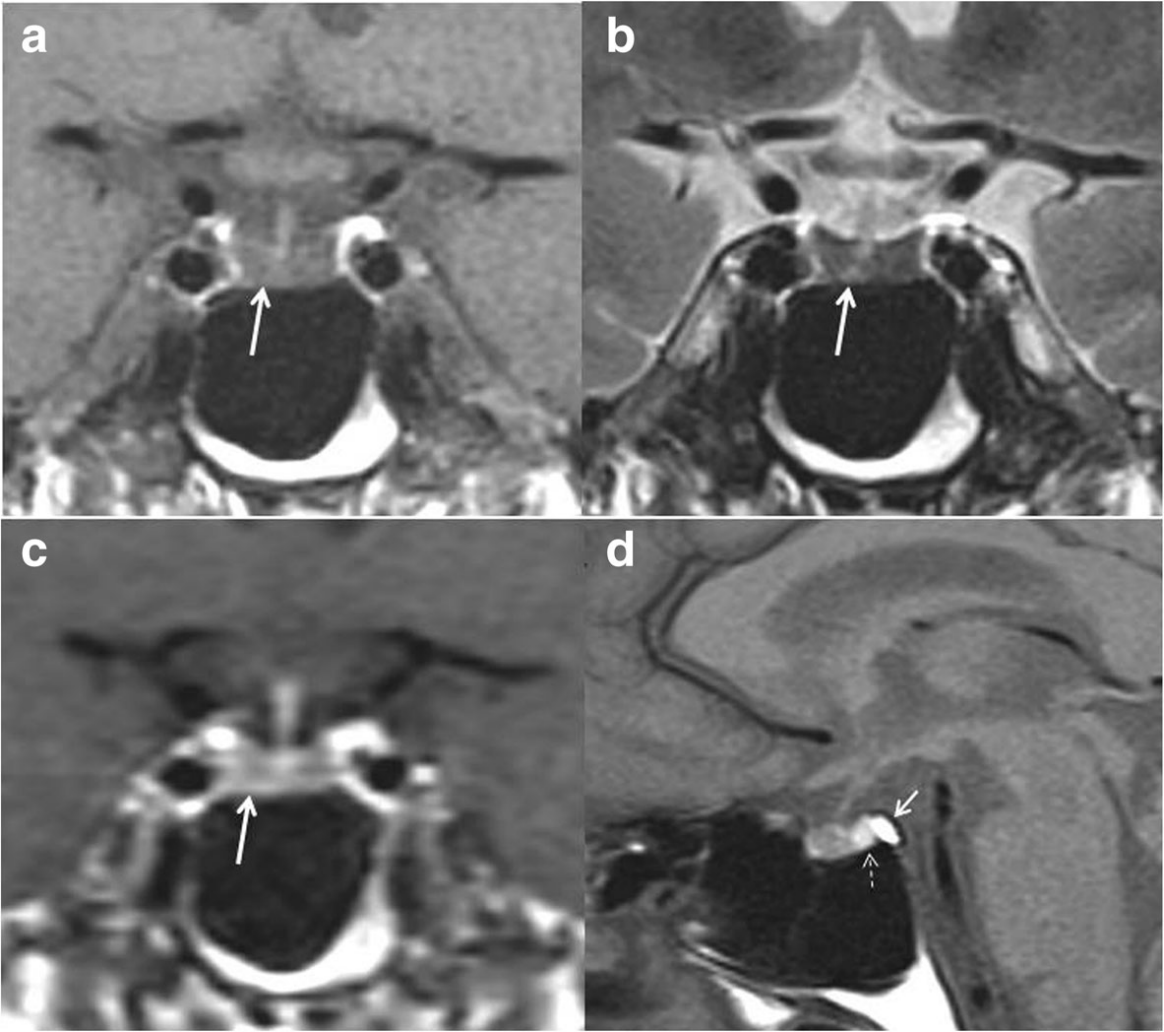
Pituitary magnetic resonance imaging performed at diagnosis. a Unenhanced coronal T1-weighted view showing low-intermediate signal intensity of the anterior pituitary gland (*arrow*) as compared to normal tissue. b Coronal T2-weighted view showing significantly decreased signal intensity of the anterior pituitary gland (*arrow*) when compared to normal. c Early coronal T1-weighted view after intravenous bolus contrast injection showing diffusely decreased enhancement of the pituitary gland (*arrow*). d Unenhanced sagittal T1-weighted view showing a small anterior pituitary gland of low-intermediate signal intensity, a normal posterior lobe displaying typical hyperintense signal (*thin arrow*), which should not be confused with the very bright signal of fatty bone marrow in the dorsum sellae (*larger arrow*). The pituitary stalk is normal
